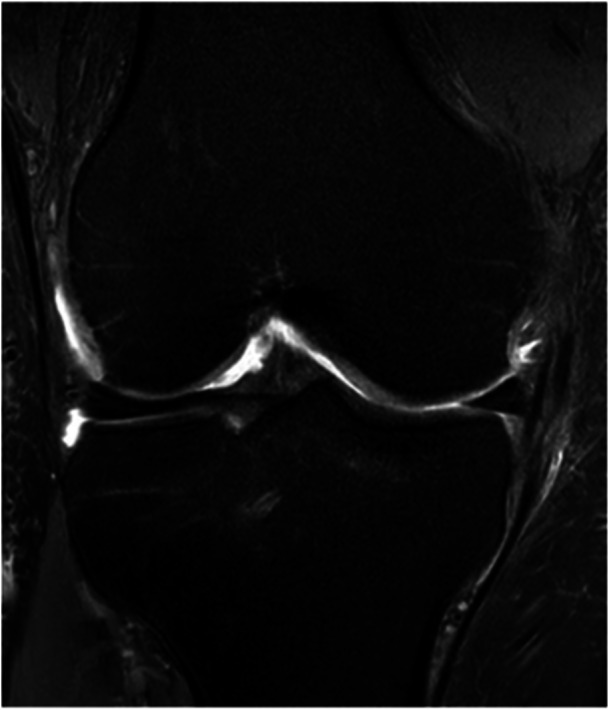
MRI coronal view of a right knee showing a paraphysiological meniscal extrusion. The MRI was performed for patellofemoral pathology and medial meniscal extrusion was detected in an asymptomatic medial compartment. The abnormality of the meniscotibial ligament at this level was detected. MRI, magnetic resonance imaging.
